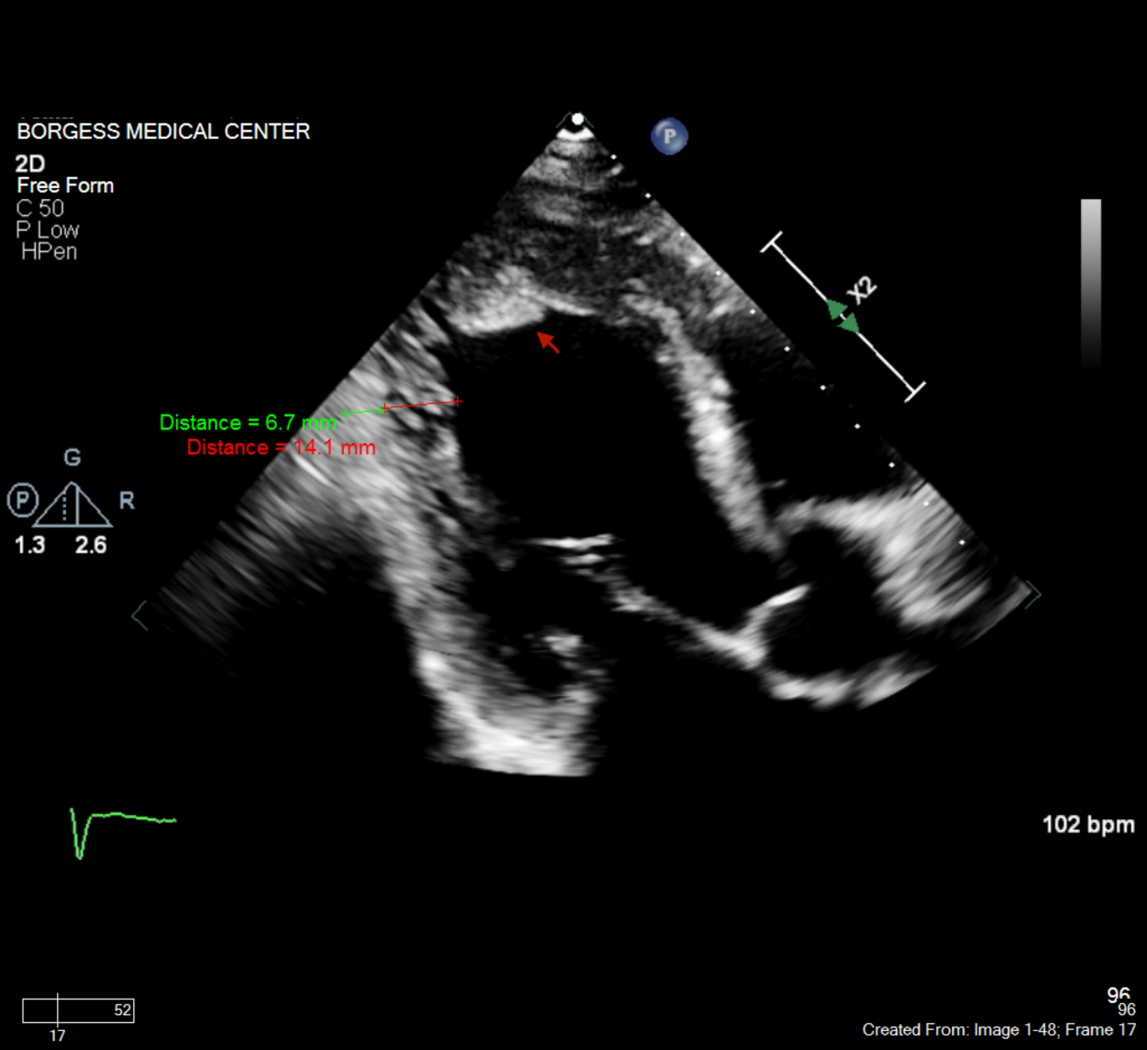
Transthoracic echocardiogram (TTE) showing left ventricular noncompaction with ratio of noncompacted to compacted myocardium of 2.1 The arrow depicts left ventricular thrombus.
